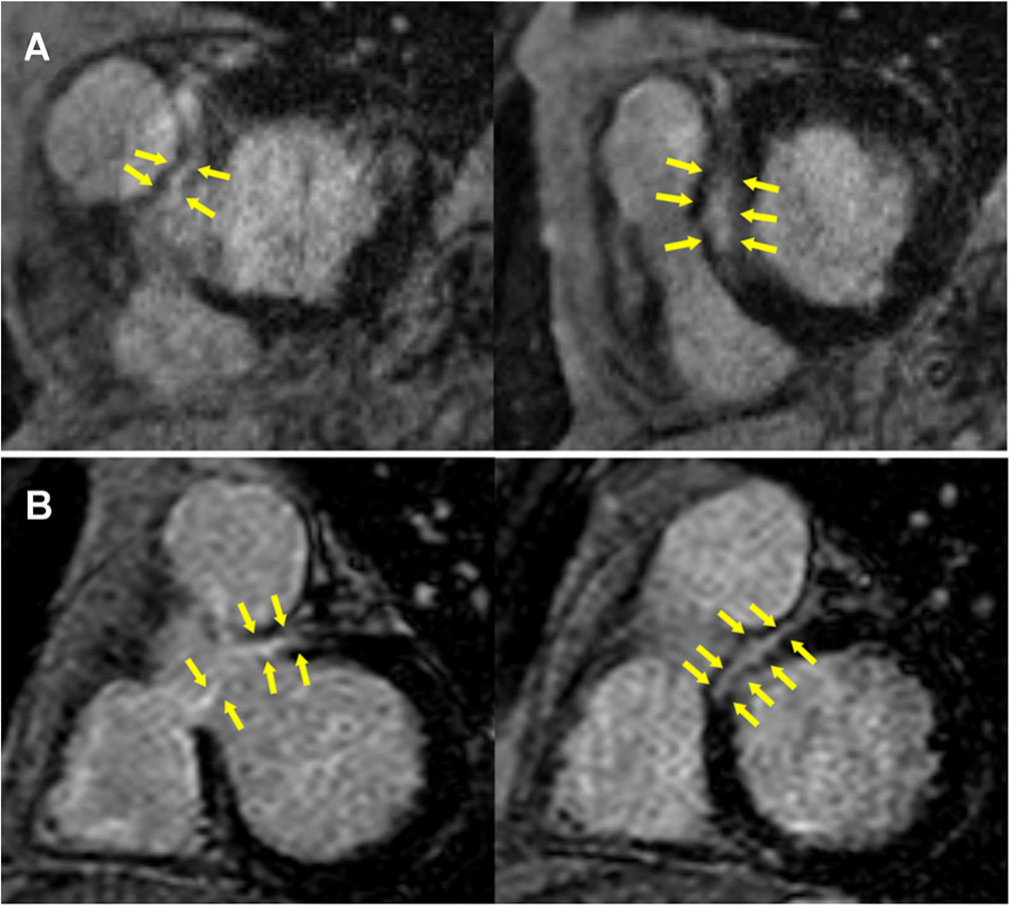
Preprocedure late gadolinium cardiac enhanced magnetic resonance imaging. Preprocedural imaging was obtained in all patients. Short axis slices are shown in two patients (Panel A and B) demonstrating intramural scar (yellow arrows) in the basal anteroseptal segments in the parahisian region extending toward the outflow tract. These patients had parahisian PVCs successfully ablated from within the sinus of Valsalva (Figures 2 and 3).
